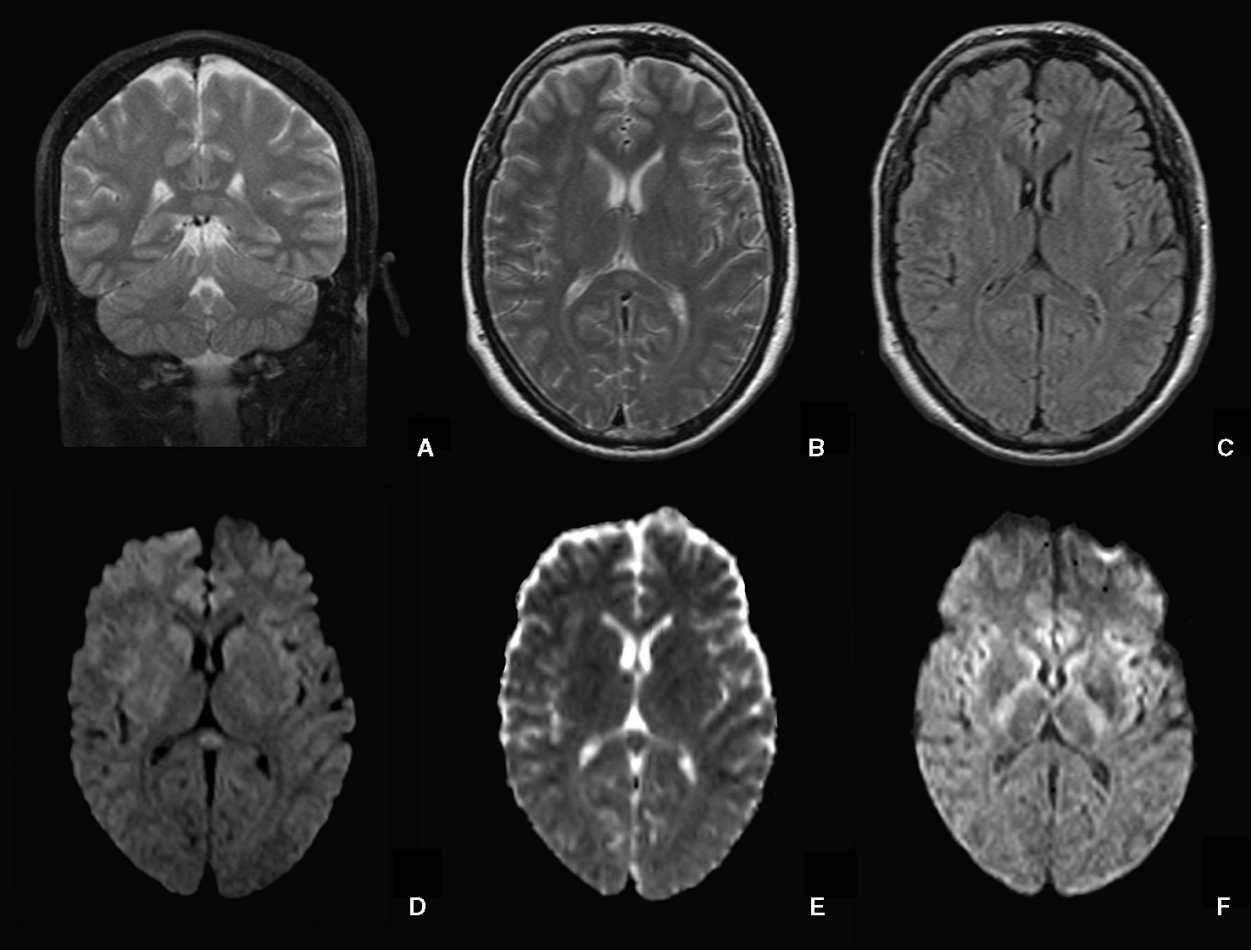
Transient focal lesion in the SCC. Coronal and axial T2-weighted images (A, B) and axial FLAIR (C) showed an oval hyperintense focal lesion in the SCC. On axial DWI (D) the lesion is hyperintense with low values on the ADC map (E). After 2 weeks, the lesion is no longer detectable on DWI (F).
